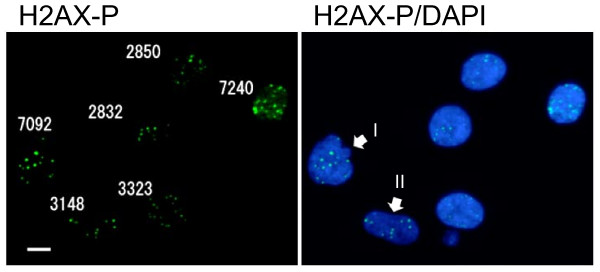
Representative SOID measurements in G2 cells. The SOID values were examined in cells 2 hours after exposure to 0.4 Gy of X-rays. The SOID was measured as described in Methods. Left panel: the SOID values in each nucleus. Right panel: white arrows indicate two nuclei with similar foci numbers but different the SOID values.
